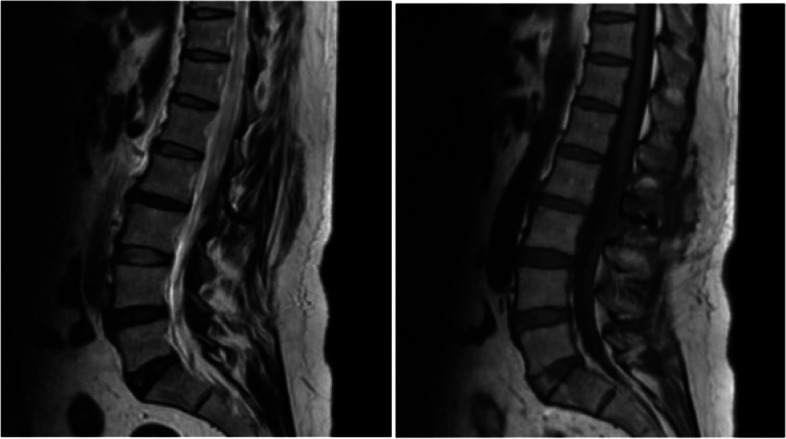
Six months after operation, lumbar MRI showed that the ligaments were repaired and healed, and there was no intraspinal scar adhesions or restenosis
